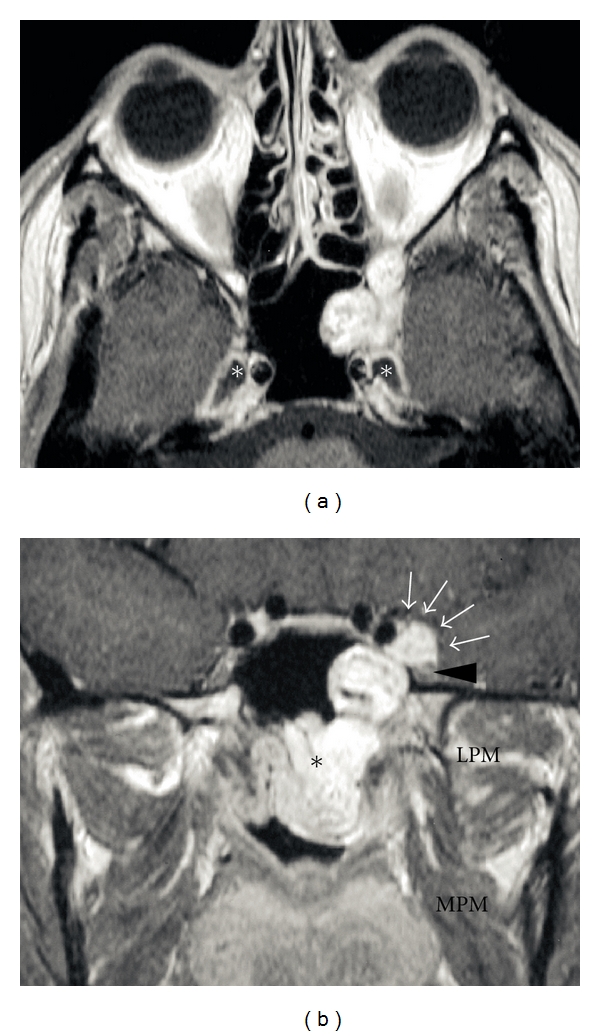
Axial (a) and coronal (b) contrast-enhanced MRI. JA with its epicenter into the root of the left pterygoid process. The nasopharyngeal component with submucosal spread is clearly evident (black asterisk). The lesion reaches the intracranial extradural compartment through the inferior and superior orbital fissures (white arrows), inferolaterally displacing the maxillary nerve (black arrowhead). The white asterisk indicates Meckel's cave. LPM: lateral pterygoid muscle; MPM: medial pterygoid muscle.
